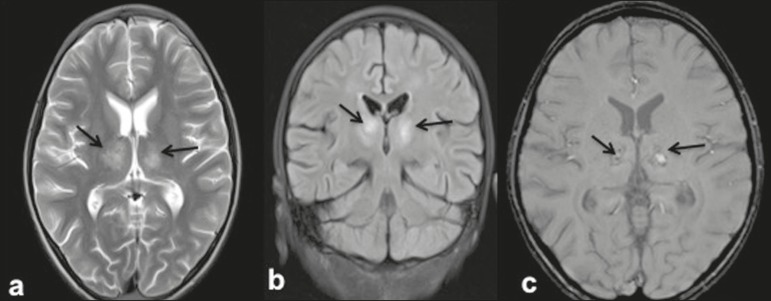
9-year-old female with dengue encephalitis. Axial T2-weighted image (a)
